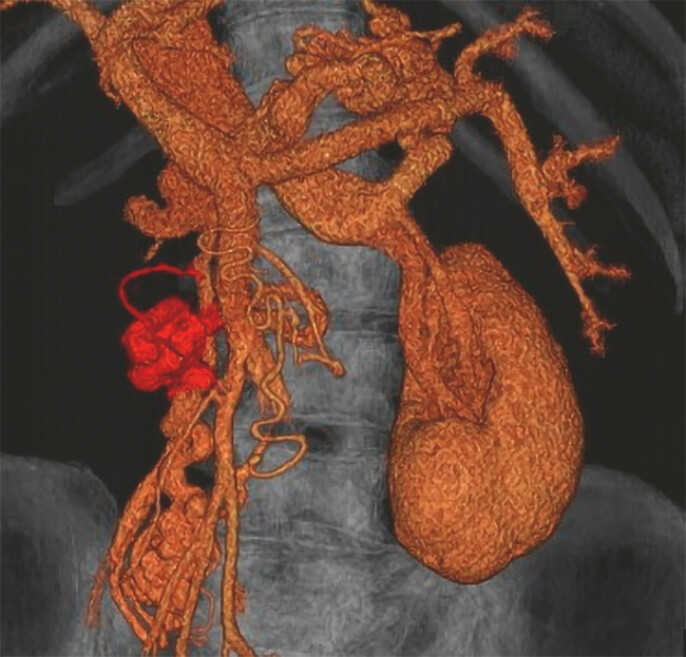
3D-CT showed duodenal varix hemodynamics that fed from the mesenteric vein to the right testicular vein.
